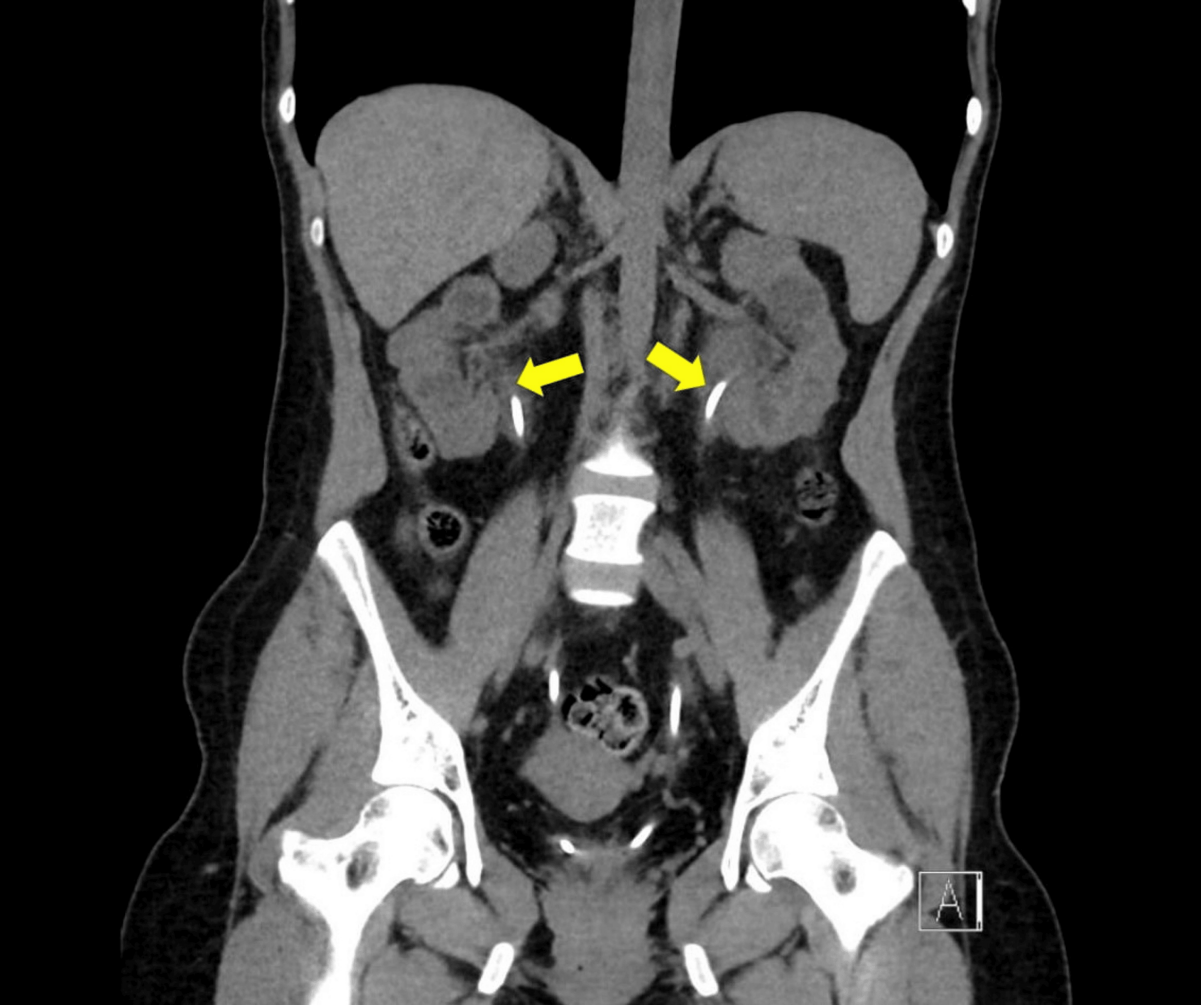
A coronal view of CT abdomen and pelvis without IV contrast showing the resolution of obstruction after placement of ureteral stents.
